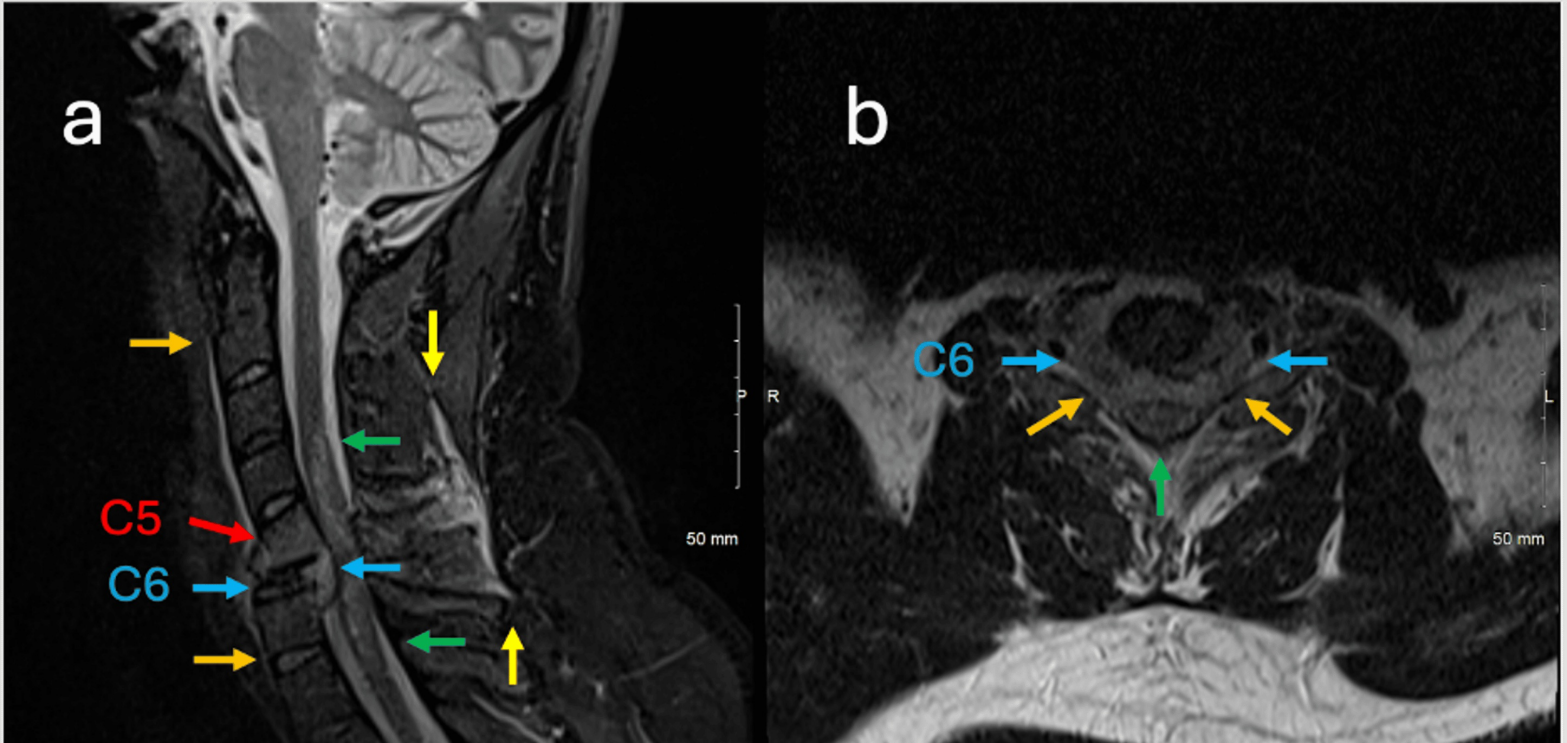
Sagittal STIR and Axial T2 MRI demonstrating metastatic paraganglioma with cervical vertebral collapse, ligamentous injury, and spinal cord compression. (a) Sagittal STIR image of the cervical spine demonstrating complete collapse of the C6 vertebral body (blue arrows) secondary to metastatic paraganglioma with destructive changes and retropulsion of the posterior vertebral body into the spinal canal. There is bone marrow edema from an acute anterior flexion teardrop fracture with mild retrolisthesis of C5 (red arrow). STIR bright cord signal abnormality (green arrows) from C4 down to T1 from cord impingement/compression is seen. There is also an interspinous and supraspinous ligamentous contusion secondary to the pathologic fracture (yellow arrows). Furthermore, there is a prevertebral hematoma (light orange arrows) from C2 to C7 from tearing of the anterior longitudinal ligament at the anterior inferior corner fracture of C5 (tip of red arrow). (b) Axial T2-weighted image at C5-C6 demonstrates circumferential collapse with retropulsion of tumour-infiltrated bone marrow into the spinal canal (green arrow) with cord impingement. There is also bilateral foraminal encroachment (light orange arrows).
